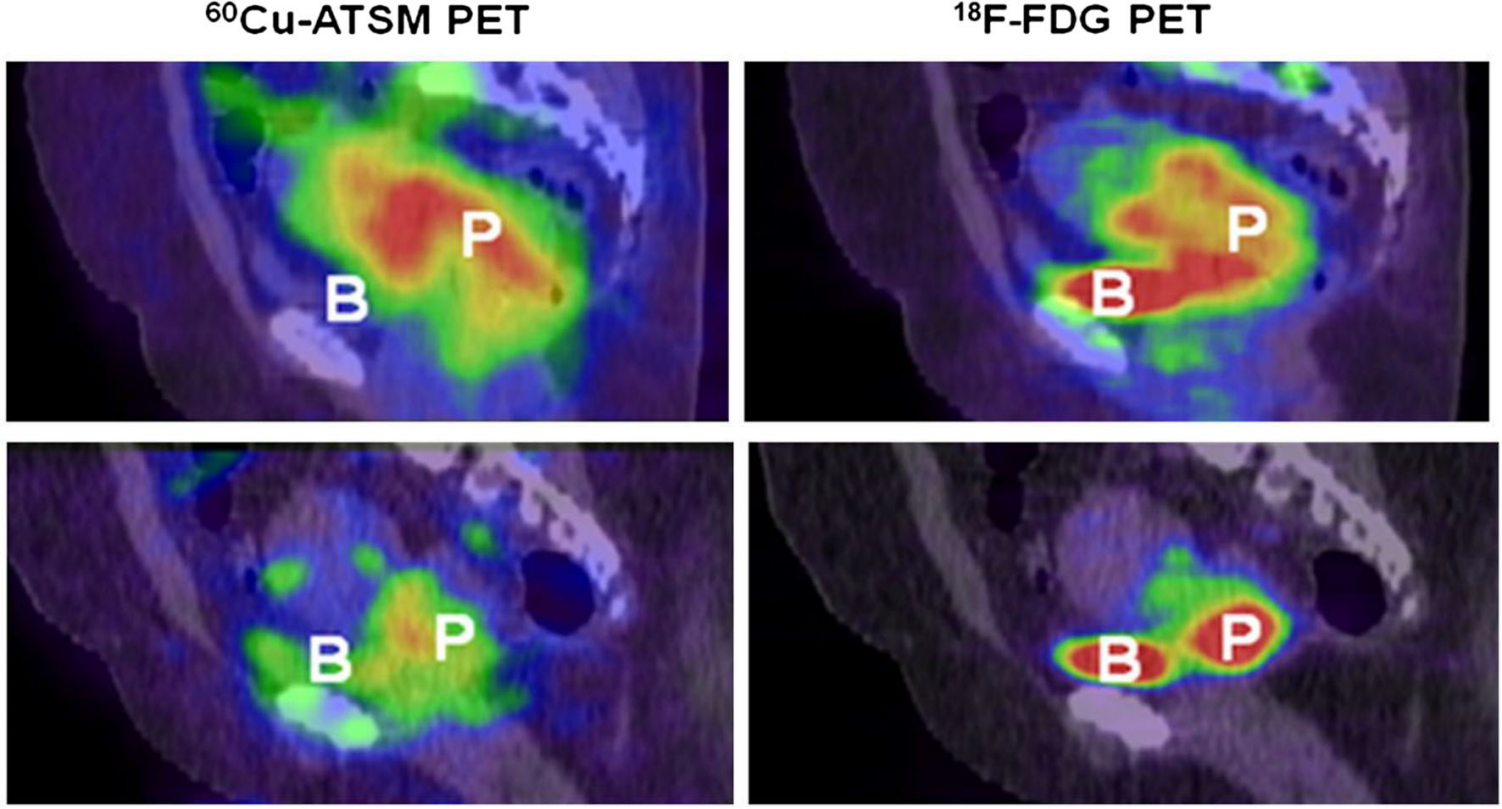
Comparison of 60CU-ATSM and 18F-FDG uptake in a hypoxic and normoxic cervix tumor. *Upper* hypoxic tumor. Sagittal 18F-FDG PET/CT image (*right*) of pelvis, showing high 18F-FDG uptake in tumor. Sagittal 60Cu-ATSM PET image coregistered with CT image (*left*) at same level, also showing high tumor uptake of this tracer (*T*/*M* = 4.5). *Lower* normoxic tumor. Sagittal 18F-FDG PET/CT image (*right*) of pelvis, showing high 18F-FDG uptake in tumor. Sagittal 60Cu-ATSM PET image coregistered with CT image (*left*) at same level, showing only mildly increased tumor uptake of this tracer (*T*/*M* = 3.0). Note that there are different patterns of 18F-FDG and 60Cu-ATSM uptake in both tumors. *P* tumor, *B* bladder. This research was originally published in *JNM* [62]. © by the Society of Nuclear Medicine and Molecular Imaging, Inc
